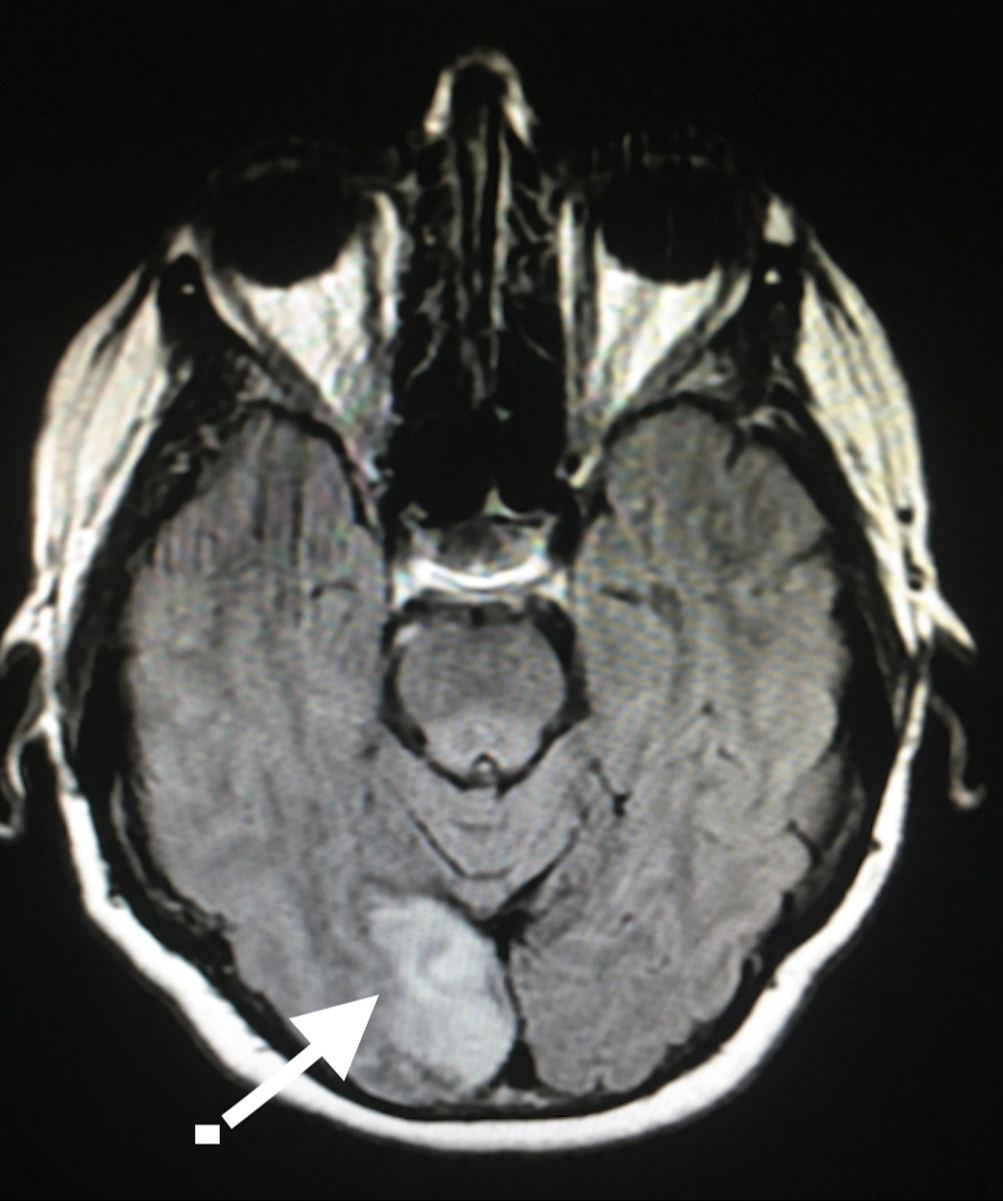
Magnetic Resonance Imaging of Brain Magnetic resonance imaging of brain without contrast demonstrating acute infarct in right medial posterior occipital lobe in the right posterior cerebral artery territory (white arrow).
